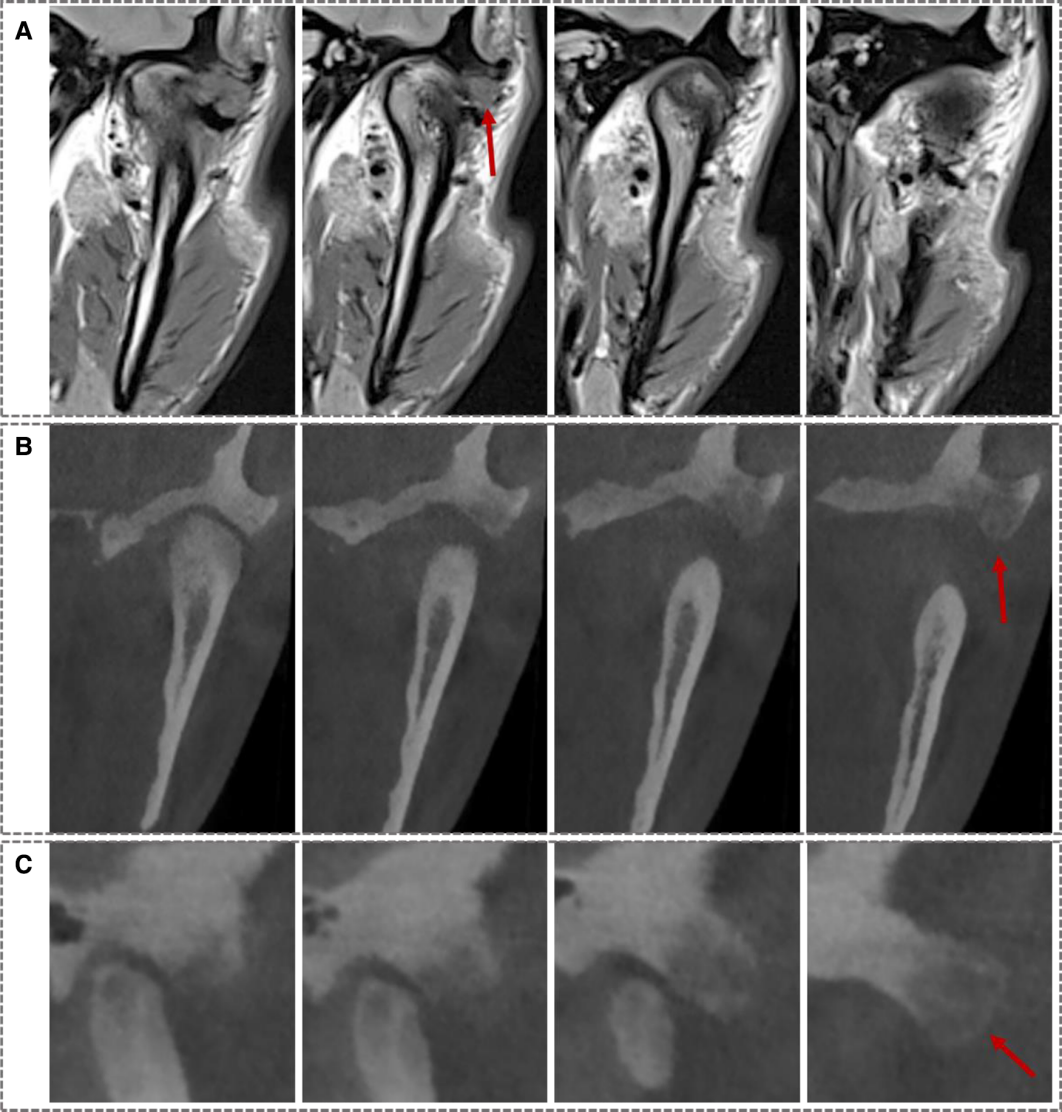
Preoperative MRI and CT images: (A) coronal MRI images showed a mass at the left articular tubercle. (B) Coronal CT images showed a heterogeneous mass at the left articular tubercle. (C) Sagittal CT images showed the same. (Red arrows indicates the lesion).
